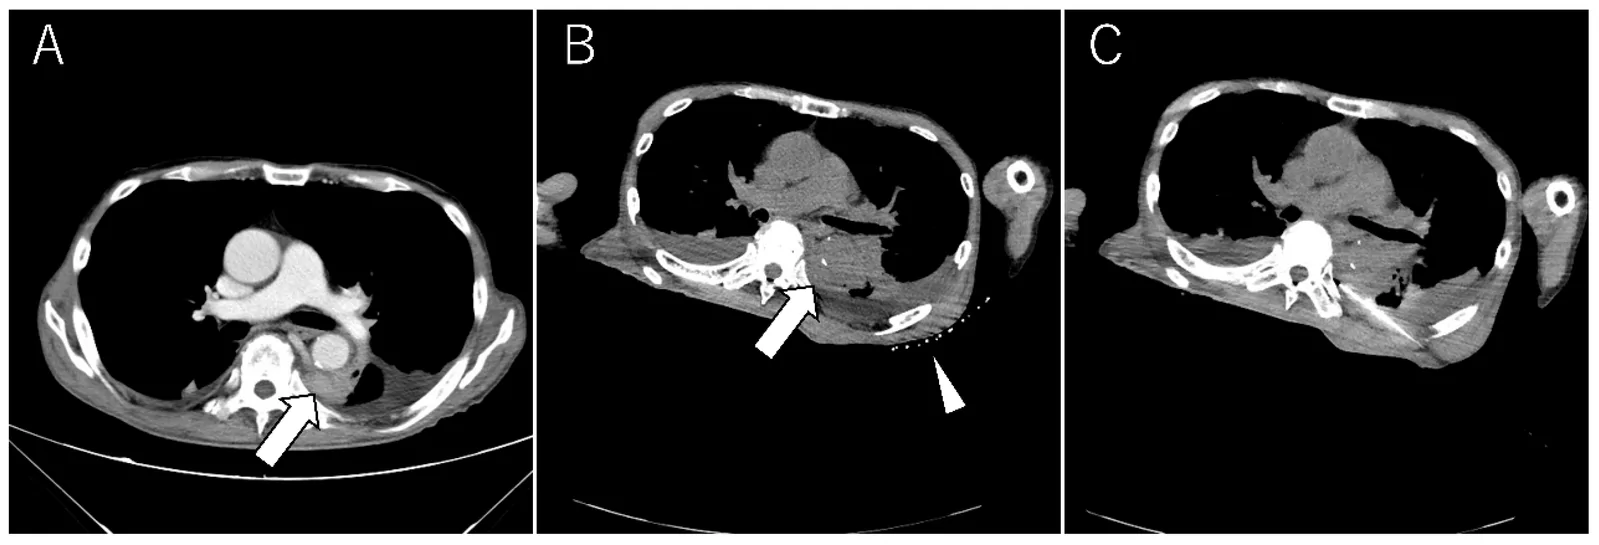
CT-guided lung biopsy using a dorsal-space mat with a posterior approach. (A) Preprocedural contrast-enhanced CT image. A soft-tissue mass (arrow) is seen posterior to the descending thoracic aorta, accompanied by pleural effusion. (B) Positioning CT image during the procedure. The patient is placed supine on the dorsal-space mat, with the puncture site marked on the back (arrowhead). The mat is highly radiolucent and therefore not clearly visible. (C) CT image during biopsy. Because the patient remains supine, the distribution of pleural effusion does not change, allowing biopsy along the planned puncture trajectory.
